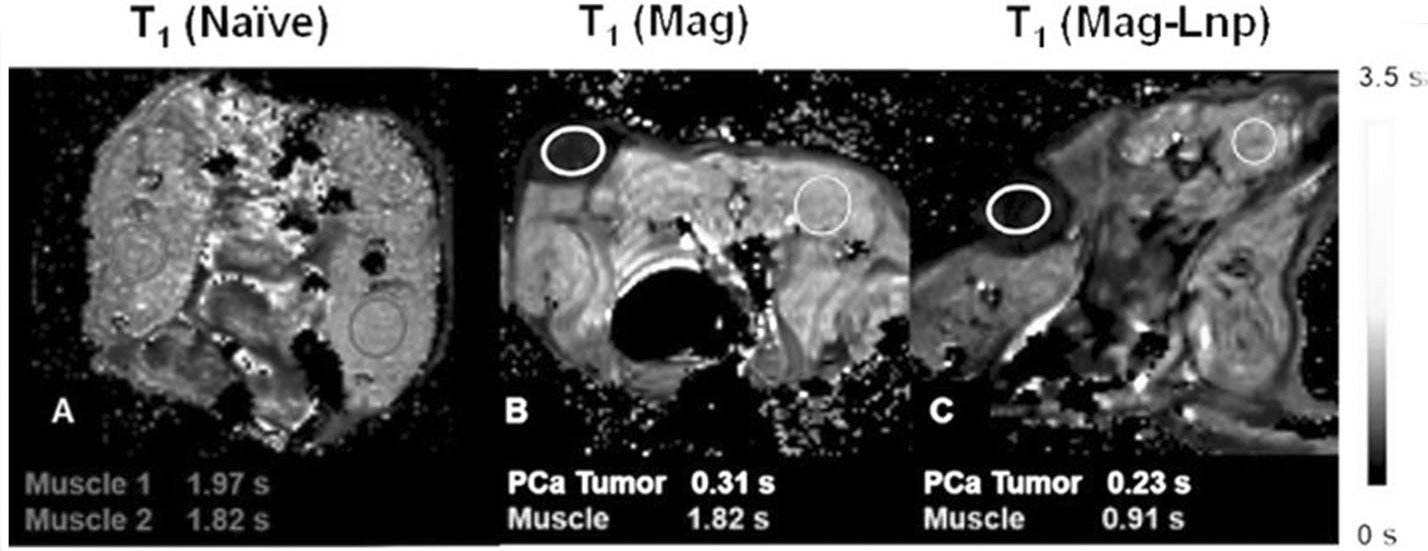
In vivo *T*_1_-maps of naïve, Mag and Mag-Lnp PCa PDX. The MR images were captured 30 min after single bolus intravenous administration Mag and Mag-Lnp. Relaxation times were extracted with ROIs in the tumor and surrounding tissue (muscles)
